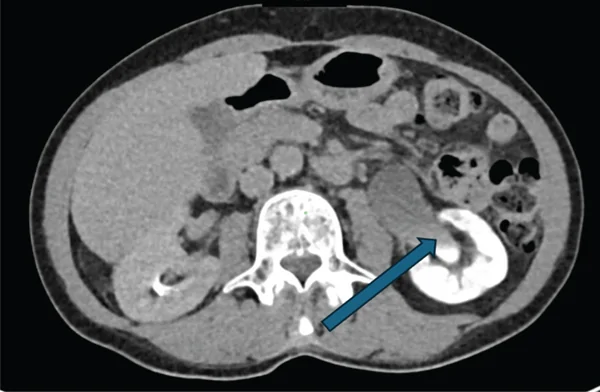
Postcontrast CT axial image demonstrating a delayed nephrogram involving the left kidney (blue arrow). A finding suggestive of high‐grade ureteric obstruction.
